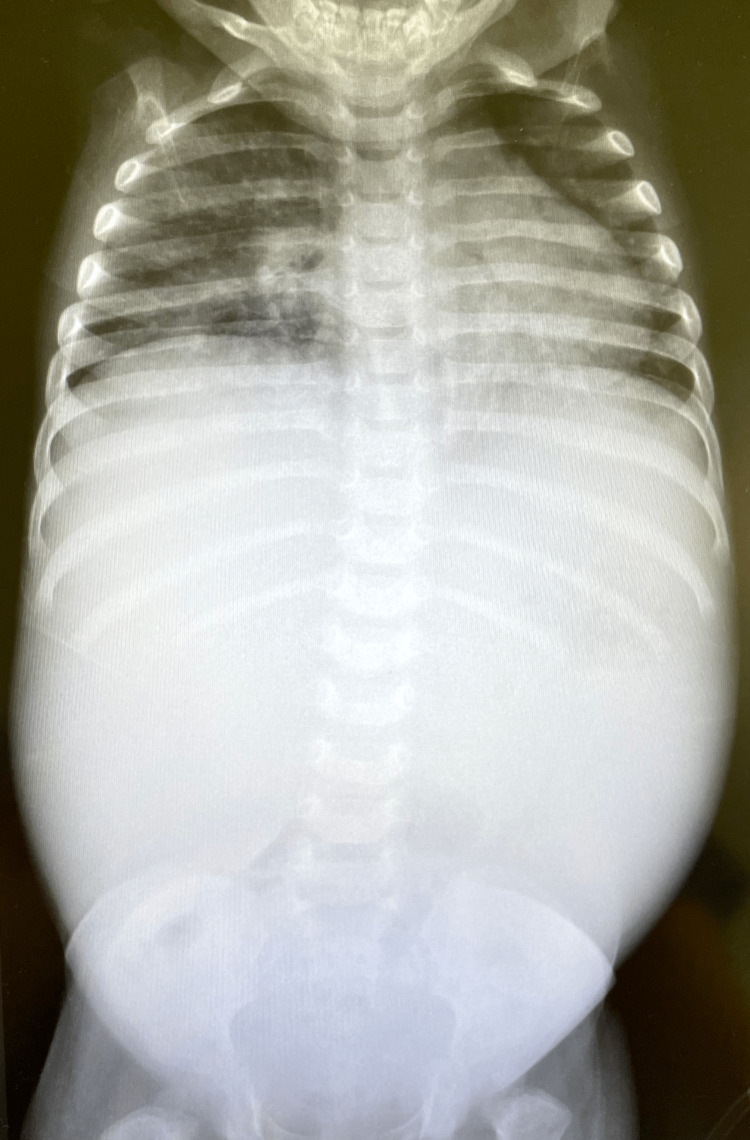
Abdominal X-ray with hepatosplenomegaly Note the radiopaque silhouette of the liver and spleen extending from the diaphragm to the iliac fossae. Note also how the thoracic cavity is reduced in size secondary to the enlarged viscera.
